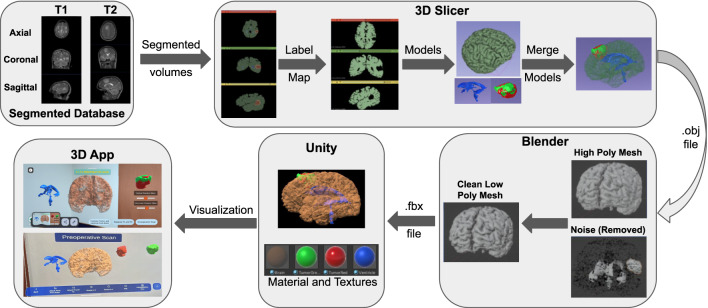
The process of XR-based 3D visualization: segmented MRI volumes from T1 and T2 scans are converted to label maps and 3D models using 3D Slicer. These models are merged, cleaned, and optimized in Blender to reduce polygon complexity. Final models are imported into Unity with textures for real-time visualization. The resulting application enables detailed AR/MR-based interactive exploration of brain anatomy and tumor data.
